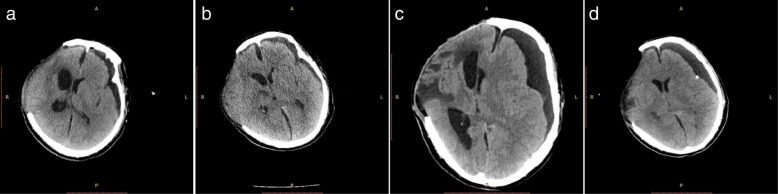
Brain CT scan after burr-hole drainage and subdural peritoneal shunt. (a) Brain CT scan on the first day after burr-hole drainage. (b) Brain CT scan after removal of the drainage tube. (c) Brain CT scan before subdural peritoneal shunt. (d) Brain CT scan after subdural peritoneal shunt
